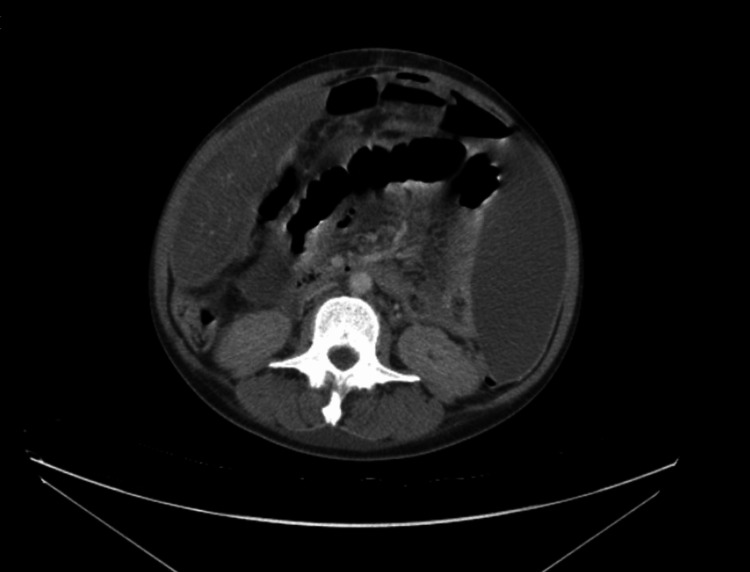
Axial CT - ascites, pneumoperitoneum, and centrally located bowel loops
